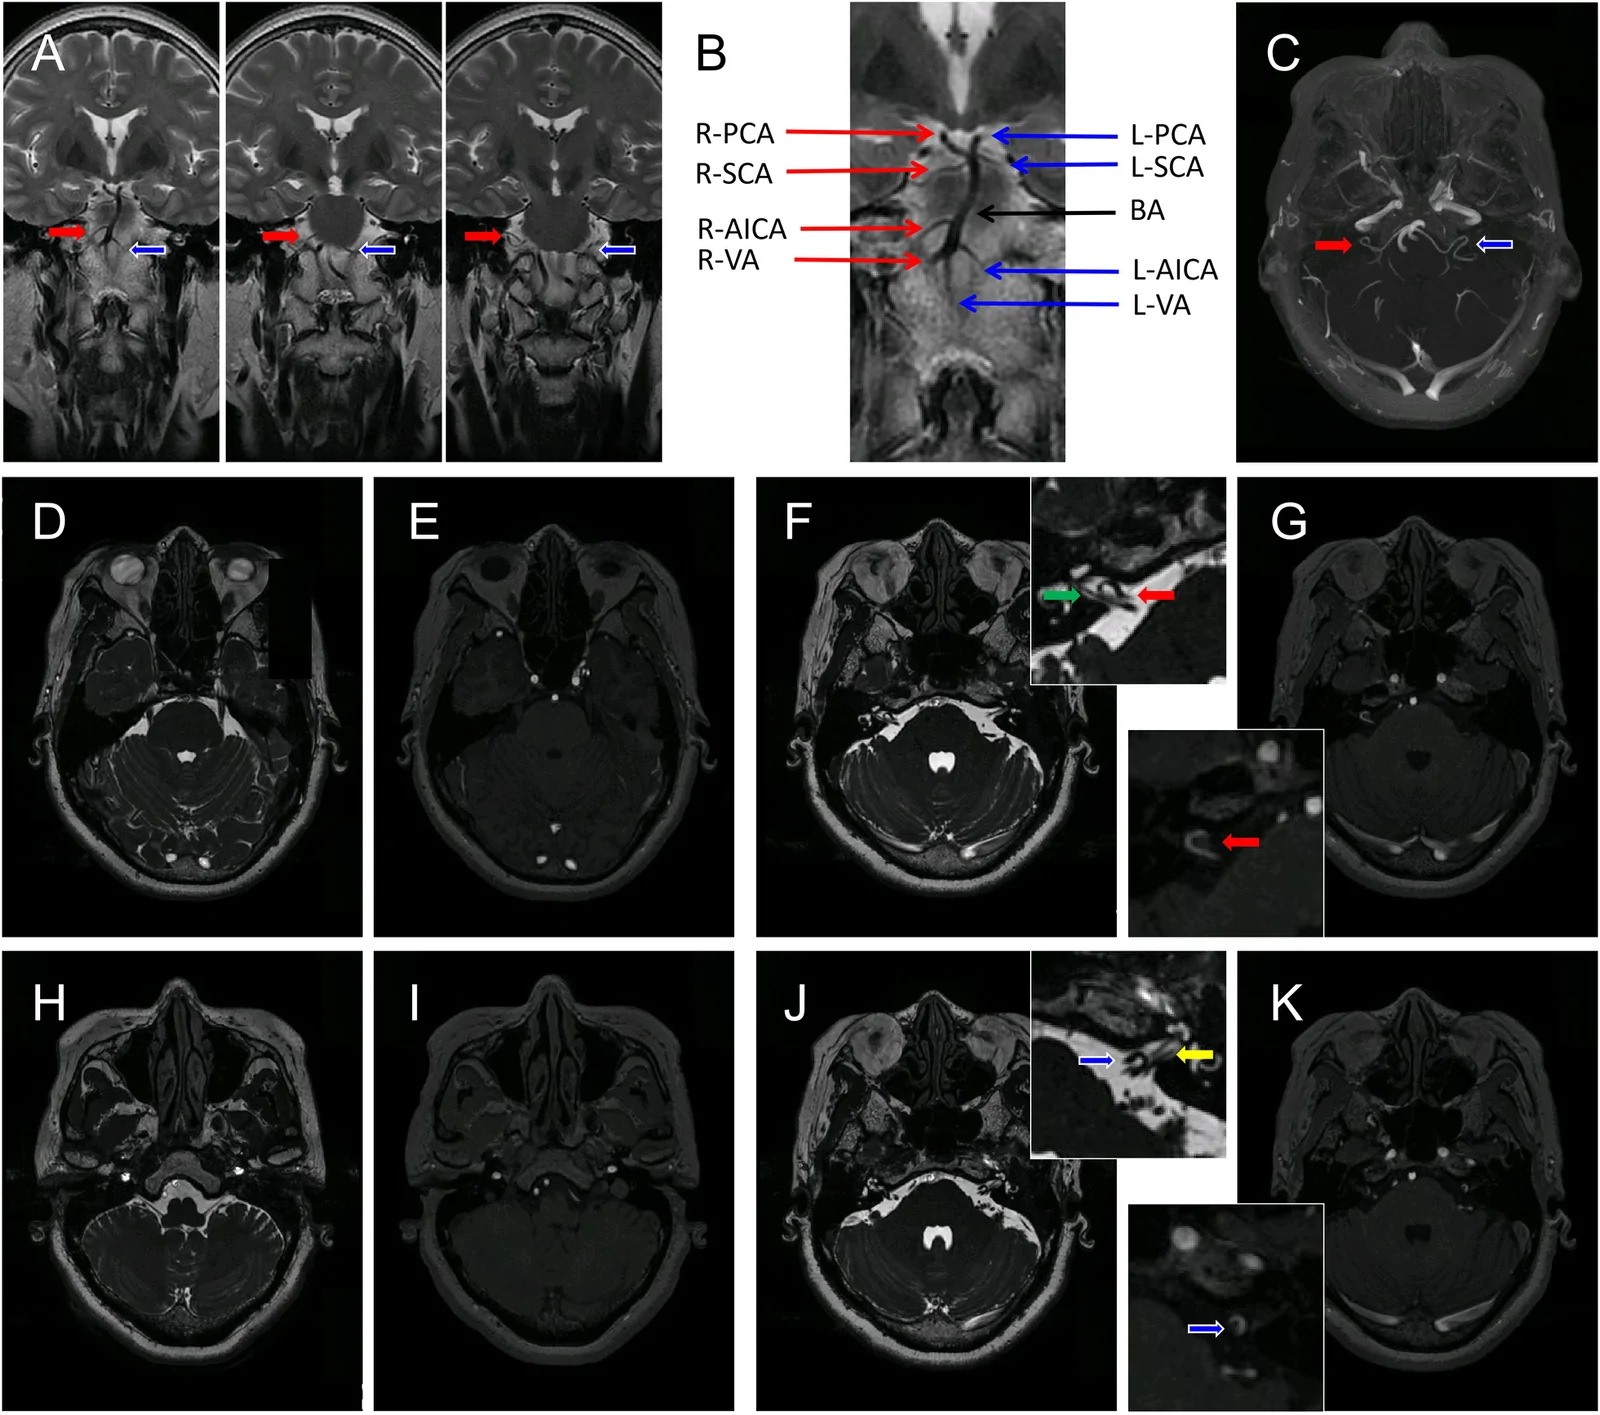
Findings of high-resolution cranial nerve MRI with ultra-thin slices. (A) Consecutive coronal thin-slice scans identified vascular loops at the cerebellopontine angle region arising from the bilateral anterior inferior cerebellar arteries (AICA). Red arrows indicate the course of the right AICA; blue arrows indicate the course of the left AICA. (B) Clear depiction of the major vascular distribution of the vertebrobasilar system at the brainstem. R-PCA, right posterior cerebral artery; L-PCA, left posterior cerebral artery; R-SCA, right superior cerebellar artery; L-SCA, left superior cerebellar artery; R-AICA, right anterior inferior cerebellar artery; L-AICA, left anterior inferior cerebellar artery; R-VA, right vertebral artery; L-VA, left vertebral artery. (C) 3D-TOF images demonstrate well-defined vascular loops formed by the bilateral AICA. (D,F) No definite vascular accompaniment or coiling is observed at the root entry zones of the trigeminal nerve. (F,G) The right AICA forms an accompanying vascular loop at the root entry zones of the right facial-vestibulocochlear nerve complex. Magnified images are placed in the upper inset [(F) 3D FIESTA-C sequence] and lower inset adjacent to the original image [(G) 3D-TOF sequence]. Red arrow indicates the right accompanying vascular loop; green arrow indicates the right facial-vestibulocochlear nerve complex. (H,I) No definite vascular accompaniment or coiling is observed at the root entry zones of the glossopharyngeal nerve. (J,K) The left AICA forms an accompanying vascular loop at the root entry zones of the left facial-vestibulocochlear nerve complex. Magnified images are placed in the upper inset [(J) 3D FIESTA-C sequence] and lower inset adjacent to the original image [(K) 3D-TOF sequence]. Blue arrow indicates the left accompanying vascular loop; yellow arrow indicates the left facial-vestibulocochlear nerve complex. FIESTA-C, fast imaging employing steady-state acquisition with cycled phases; 3D TOF, three-dimensional time-of-flight.
